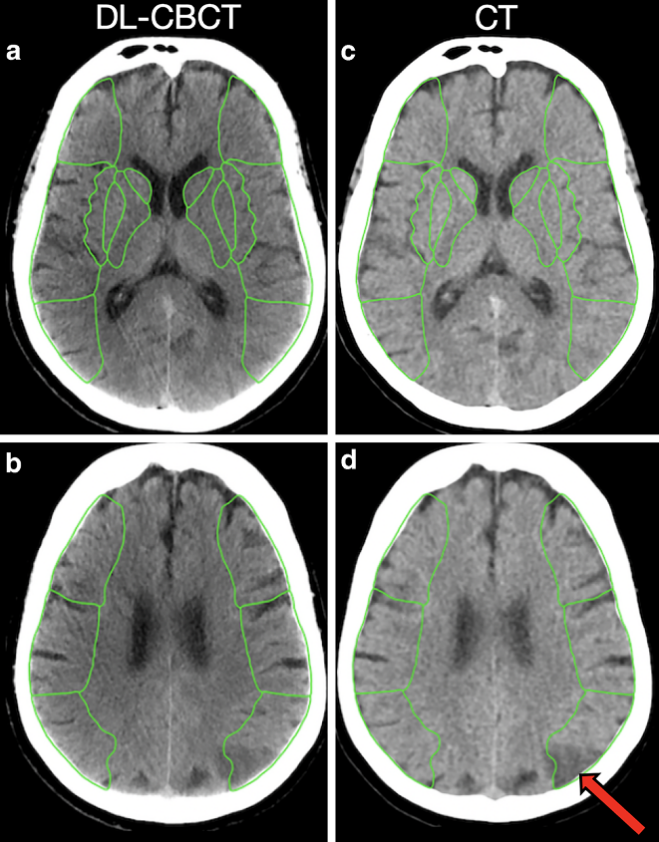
Axial non-contrast images of a participant with ischemic stroke. a–d shows ASPECTS regions boundaries at the level of the basal ganglia (a and c) and superior to the basal ganglia (b and d). a, b are DL-CBCT 75 keV images, c, d are CT images. There is an infarct in the left hemisphere M6 region (red arrow). All W/L: 75/25, 5 mm slices with average intensity projection. *DL-CBCT* Dual-layer cone-beam CT
